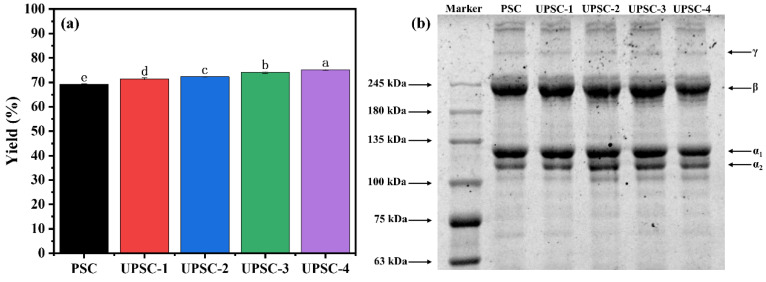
The yield (a) and SDS-PAGE profiles (b) of pepsin-soluble collagen-I from albacore skin extracted at different ultrasonic powers (non-ultrasonic treatment, PSC; 150 W, UPSC-1; 300 W, UPSC-2; 450 W, UPSC-3; 600 W, UPSC-4). Note: different letters in (a) indicate significant differences (*p* < 0.05).
